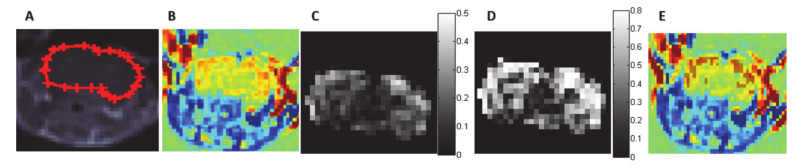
Examples of calculated maps: (A) A T1-MRI slice with the plotted brain region of interest (ROI) overlaid. (B) Treatment response assessment maps (TRAMs) calculated by subtracting the 1st series post-contrast from the series obtained 30 min post-contrast injection showing red (contrast accumulation) regions (demonstrating higher blood–brain barrier disruption (BBBd) in the cortex). (C) Maximal intensity increase map in the same brain slice. (D) r^2^ map depicting the fit r^2^ of each pixel in the same brain slice. (E) Pixels included in the BBBd ROI (intensity increase > 5% and r^2^ > 0.6), marked in brown and overlaid on the TRAMs.
